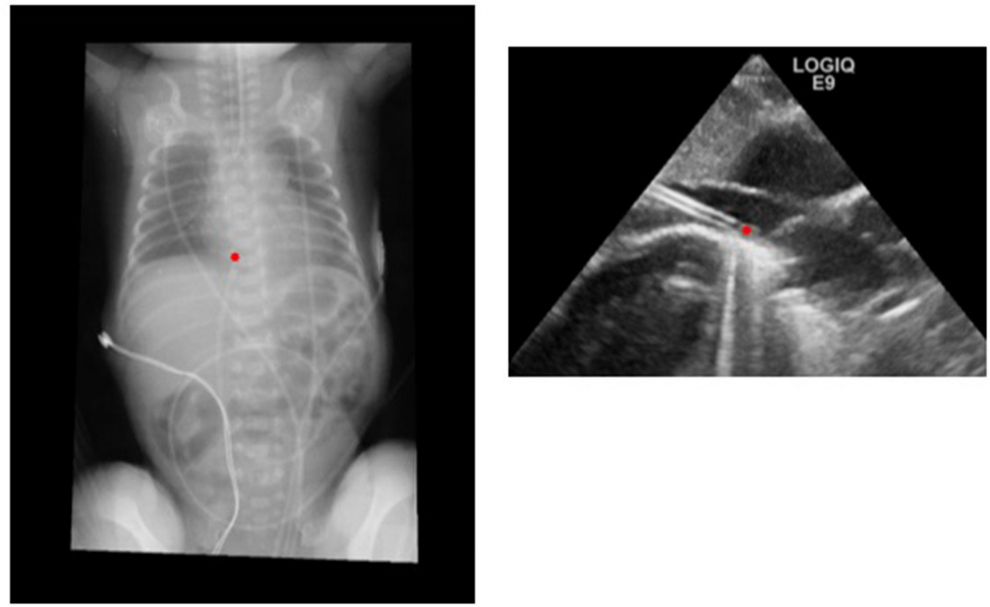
Comparison of radiographic and ultrasound images of a correctly positioned UVC.
